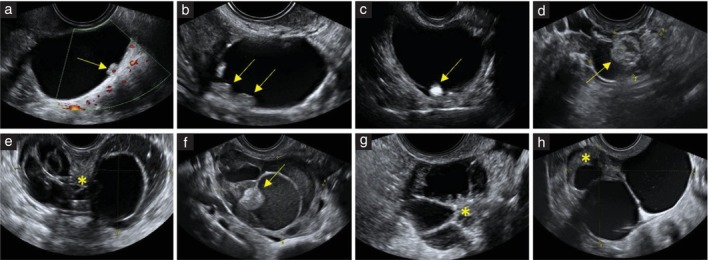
Power Doppler (a) and grayscale (b–h) ultrasound images showing less common features of serous cystadenomas. (a–c) Unilocular‐solid cysts with papillary projections (arrows). (d–h) Multilocular‐solid cysts with papillary projections (arrows) or other solid components (asterisks).
